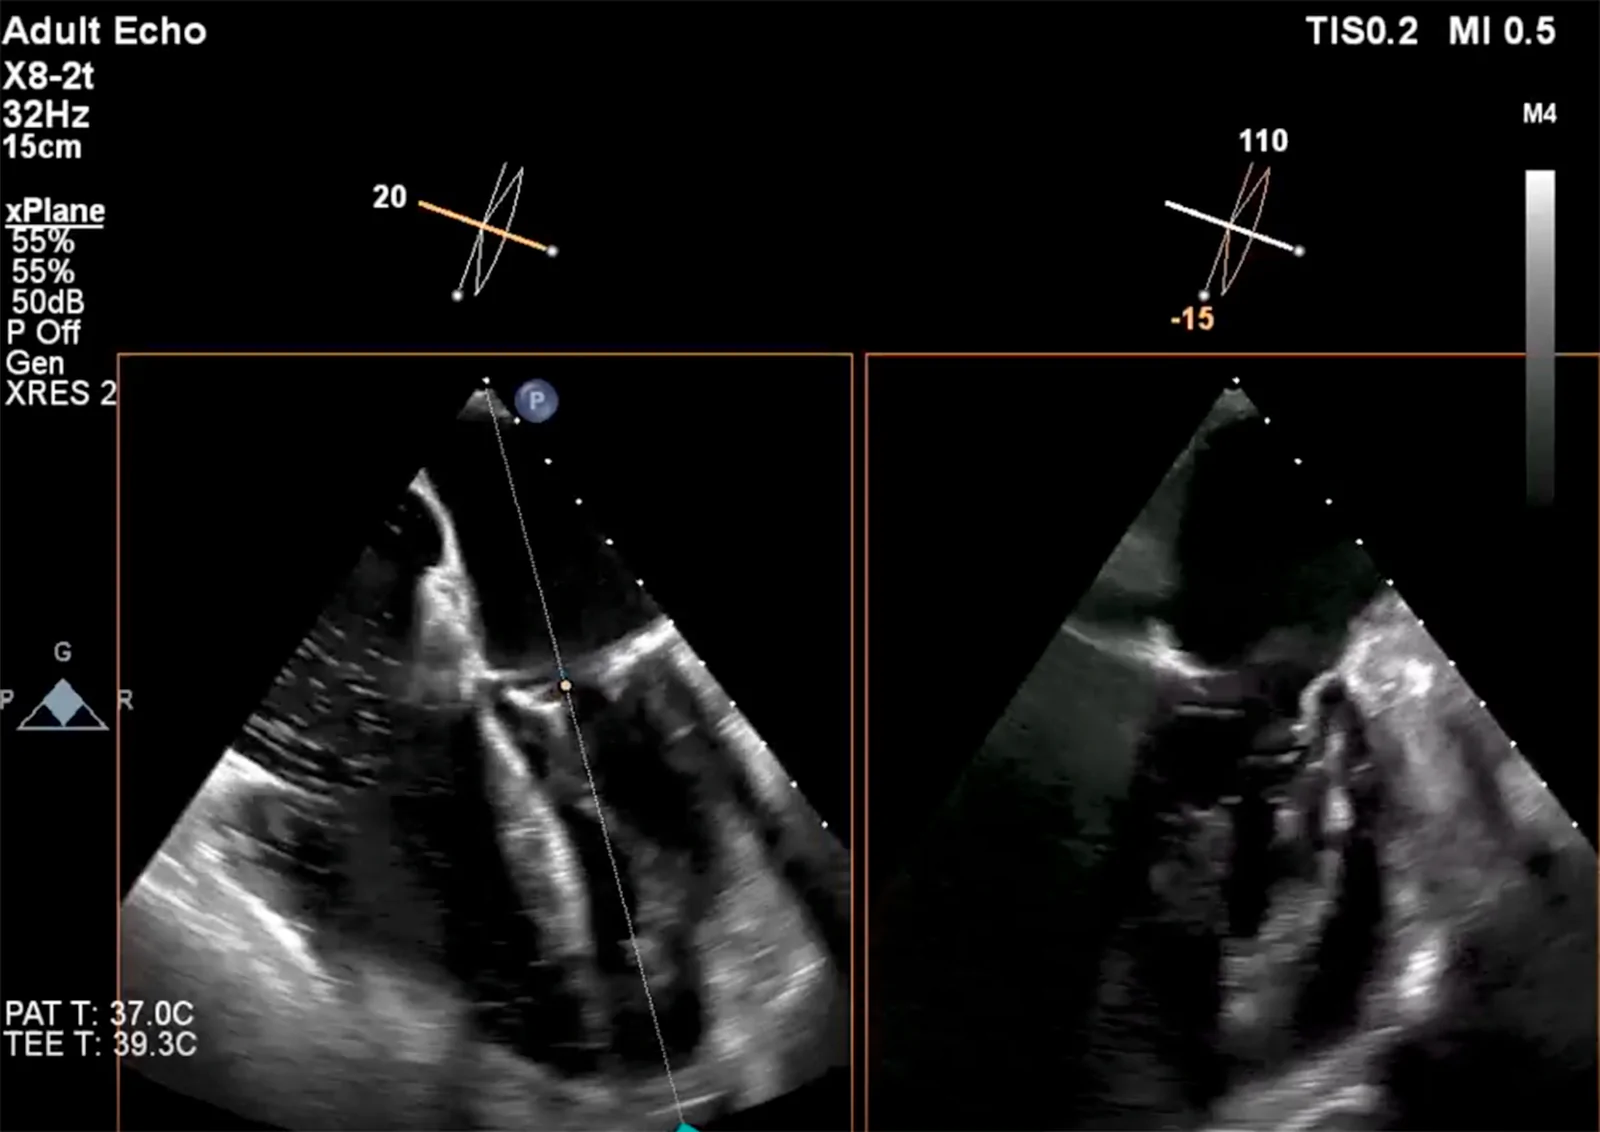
X-Plane of Midesophageal 4-Chamber on Transesophageal Echocardiography Demonstrating an Underfilled Left Ventricle and Overloaded Right Ventricle
